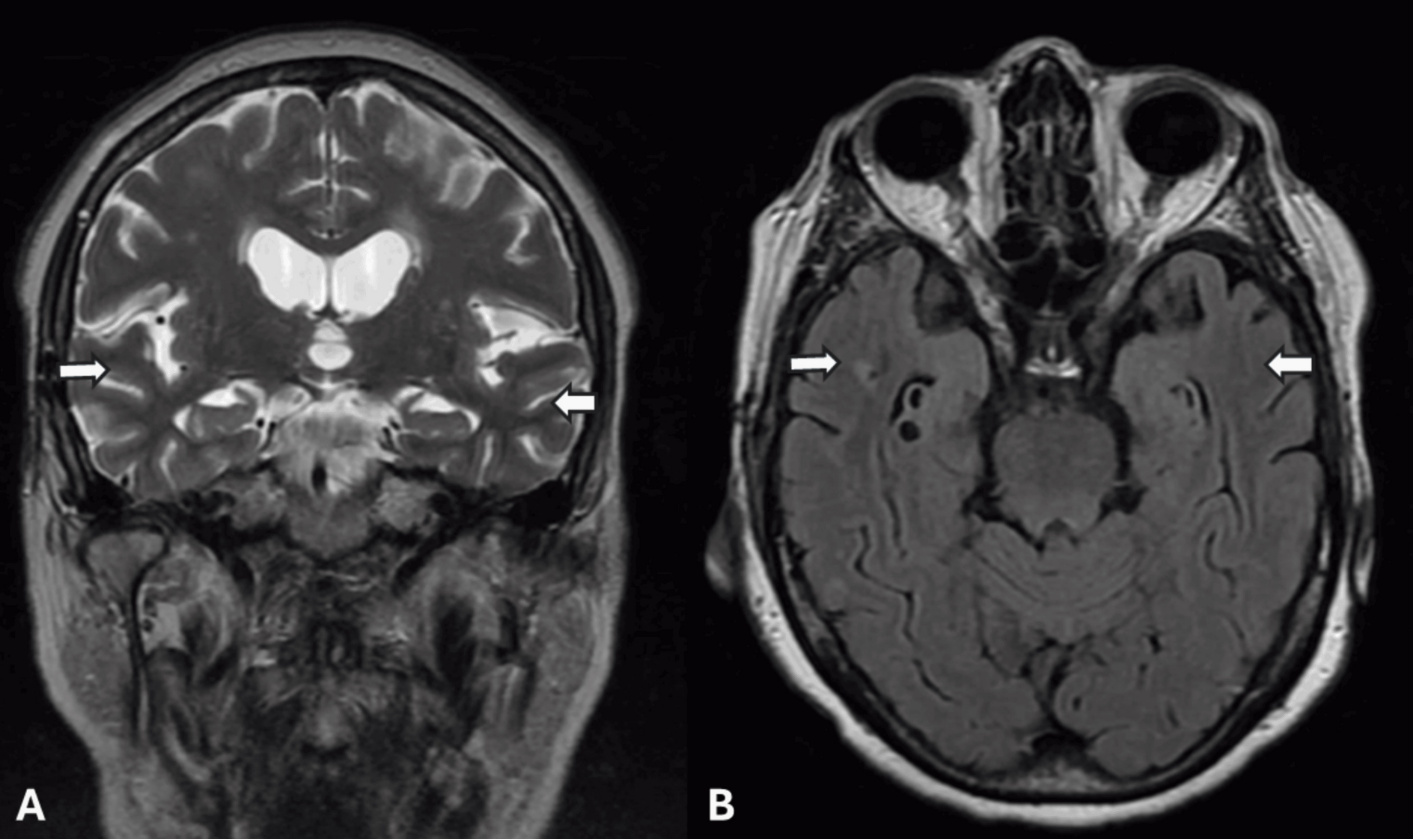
Follow-up MRI of the brain; T2 coronal (A) and T2 FLAIR (B) images demonstrating resolution of T2 FLAIR hyperintensities and vasogenic edema involving bilateral temporal lobes MRI: magnetic resonance imaging; FLAIR: fluid-attenuated inversion recovery
